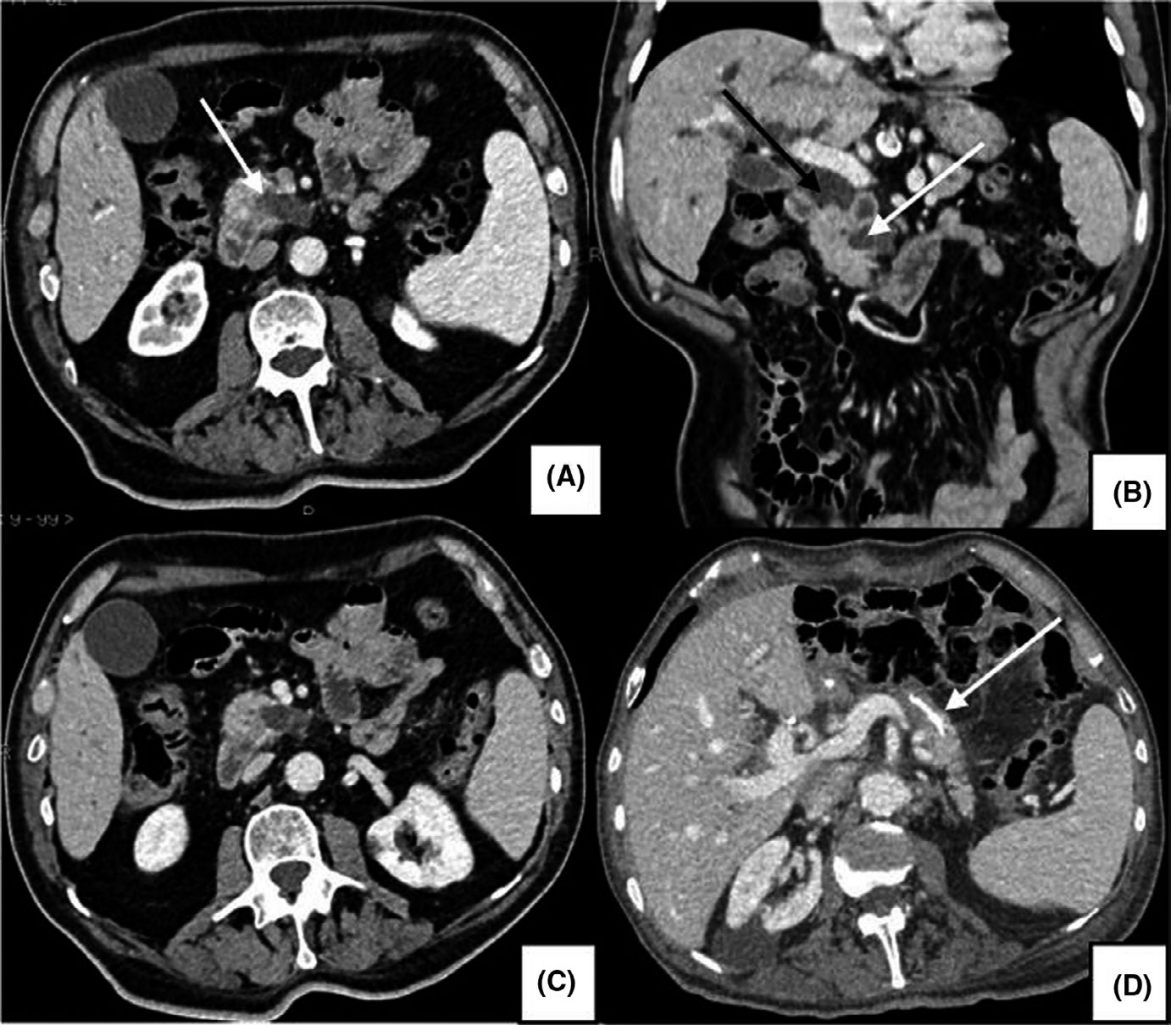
Portal phase CT scans, showing chronic pancreatitis with dilatation of the MPD and parenchymal atrophy (A, B, C). A, axial image: BD‐IPMN with typical communication between the MPD and the cystic lesion (white arrow). B, coronal image: dilatation of the extrahepatic bile duct (black arrow) and upstream dilatation of the MPD (white arrow). D, axial image: pancreatic reconstruction, in which the endo‐Wirsung catheter is visible (white arrow)
